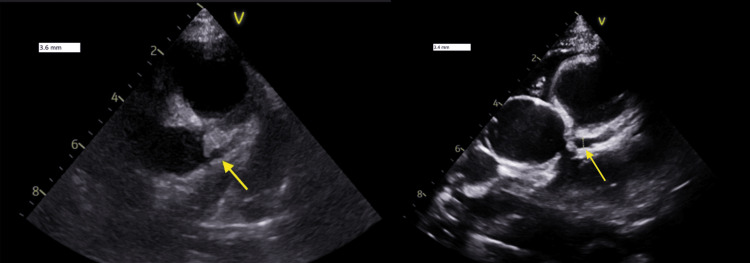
Day 5 echocardiography showing LMCA dilation (left); day 7 echocardiography showing improvement in LMCA dilation (right). Measurements were taken at end-diastole. LMCA: left main coronary artery
